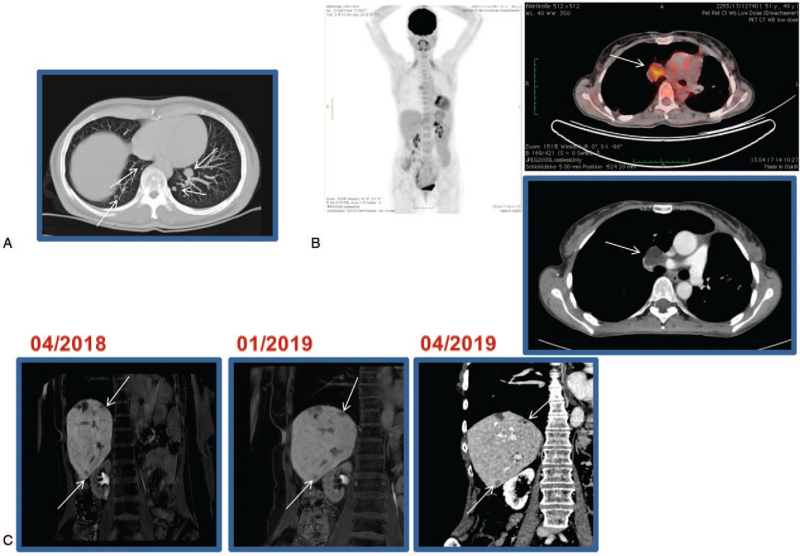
(A) CT scan of thorax dated February 1, 2010 (lung window, axial maximum intensity projection) shows high level suspicion of at least 7 lung metastases on both sides. (B) FDG-PET/CT scan dated April 13, 2017 shows moderately intensive FDG image of the known right hilar lymph node metastasis and CT scan of thorax/abdomen from February 1, 2017 shows progressive size right hilar lymph node metastasis. (C) CT scan of abdomen and MRI of liver from April 2018, January and April 2019 show constancy or low regression of HCC-suspicious lesions in liver segments VI and VIII. The arrows point to metastases. HCC = hepatocellular carcinoma, MRI = magnet resonance imaging.
